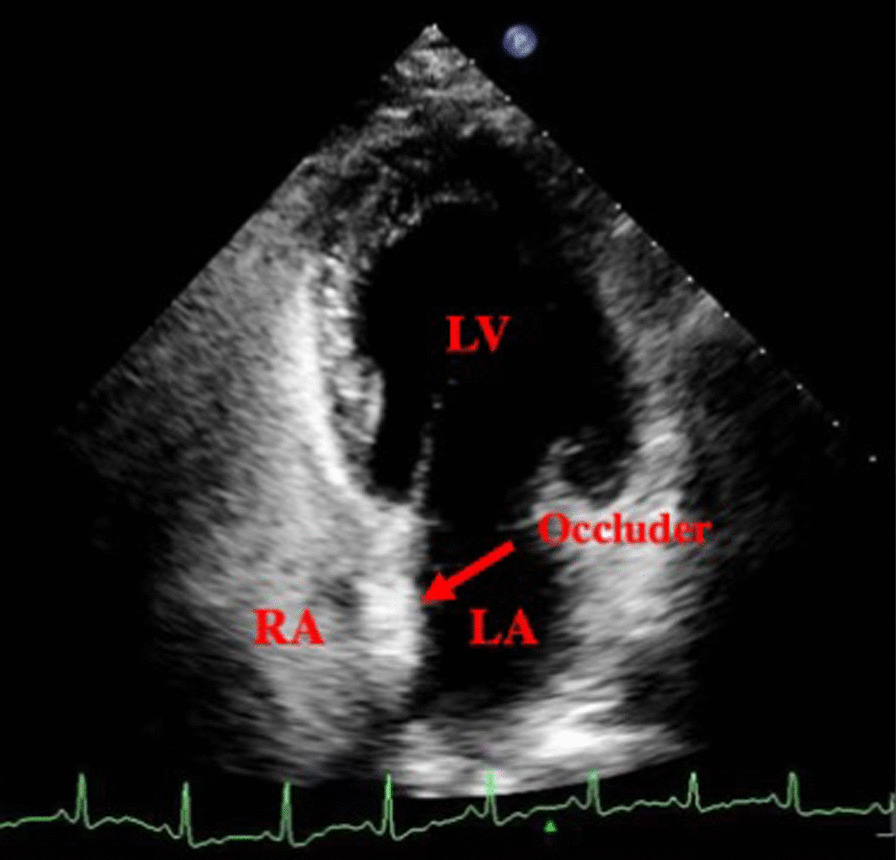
Negative transthoracic UFT result after PFO occlusion. There is no microbubble in the LA. *LV* left ventricular, *RA* right atrial, *LA* left atrial, *PFO* patent foramen ovale, *UFT* ultrasound foaming test
